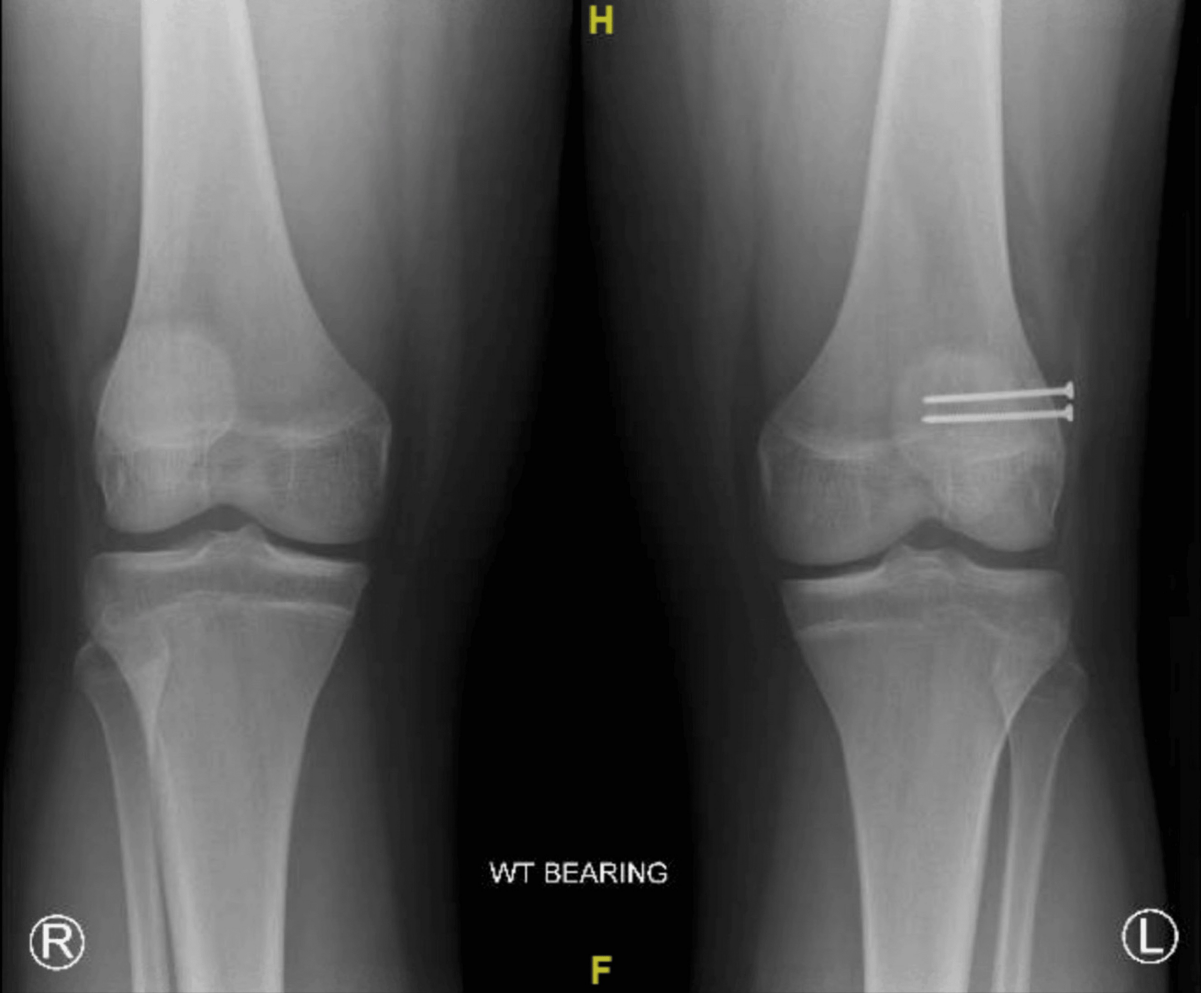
Three-month postoperative X-ray bilateral knee AP weight-bearing view AP: Anteroposterior.
